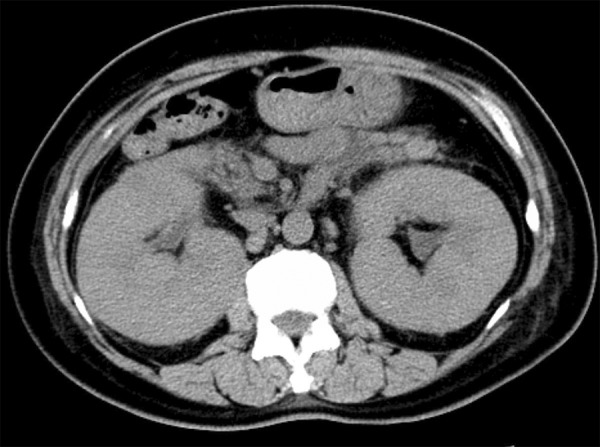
Abdominal computed tomography scan showing the bilateral enlarged kidney as well as retroperitoneal lymphadenopathy.
